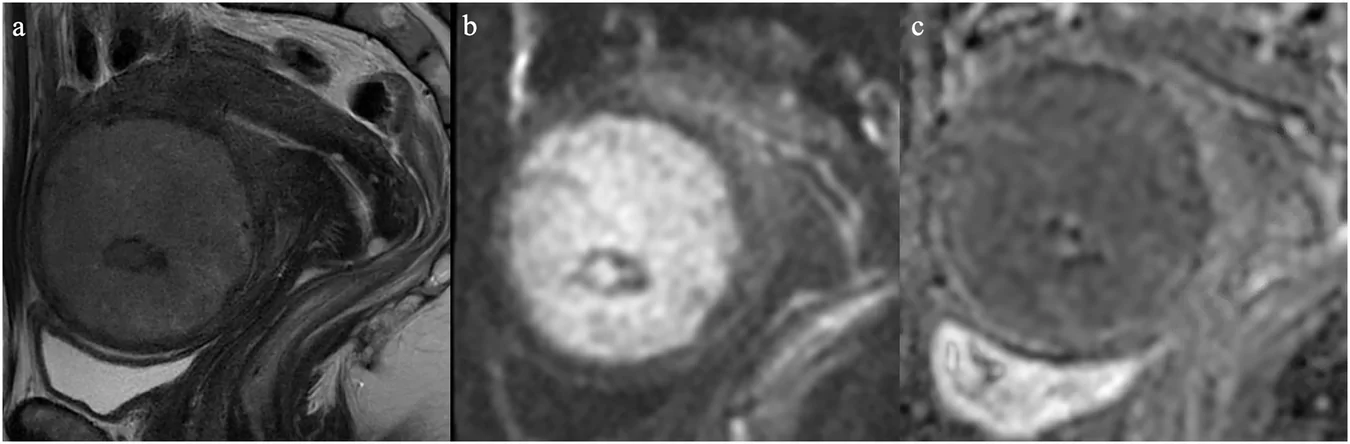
Cellular leiomyoma in a premenopausal woman. a T2-weighted sequence; b DWI sequence; c ADC MAP. The images show a myometrial lesion with intermediate T2W signal, high signal intensity, and an ADC map showing low values, measured at 0.9 × 10⁻³ mm²/s. The lesion showed homogeneous enhancement and smooth margins, allowing classification as score 3 according to Zlotykamien-Taieb et al [7]
